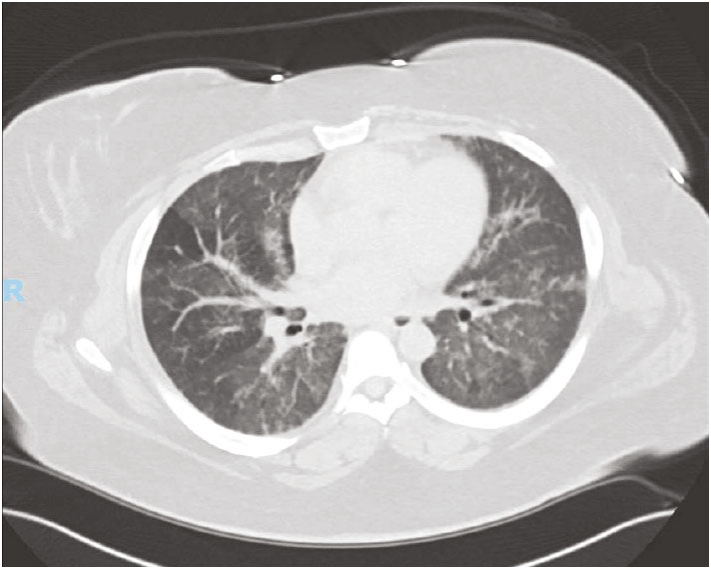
Computed tomography of the chest without contrast. Decreased ground glass opacities bilaterally, which is consistent with resolving pulmonary hemorrhage.
